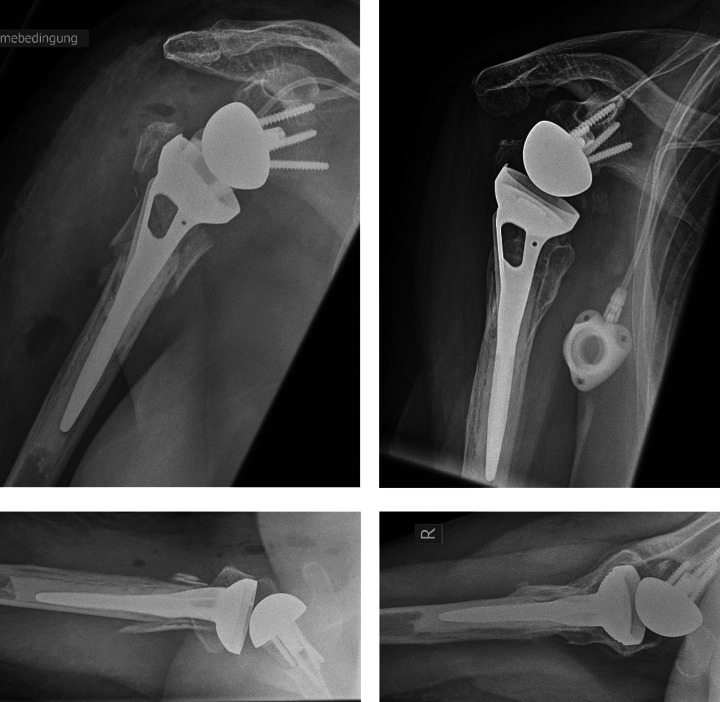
Postoperative plain radiographs in a.p. and axial radiographic view on the left side and secondary displacement of the greater tuberosity in RSA in a.p. and axial radiographic view on the right side.
